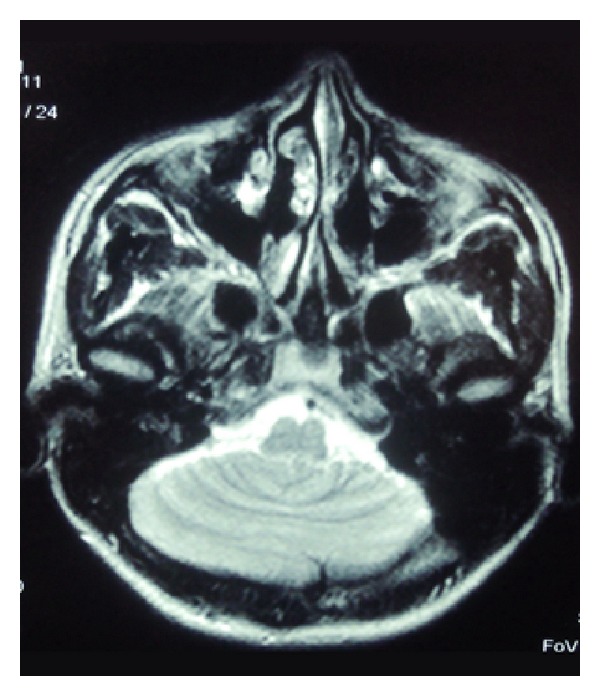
Magnetic resonance imaging of brain which demonstrated partially developed vermis and rhombencephalosynapsis.
